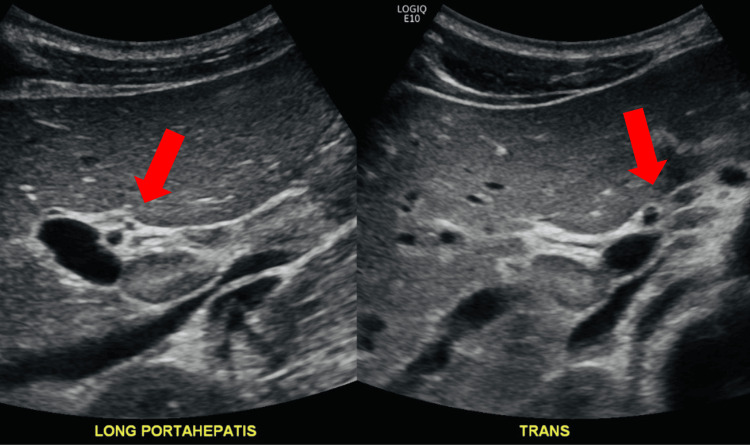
RUQ ultrasound with porta hepatitis lymph nodes RUQ: right upper quadrant
